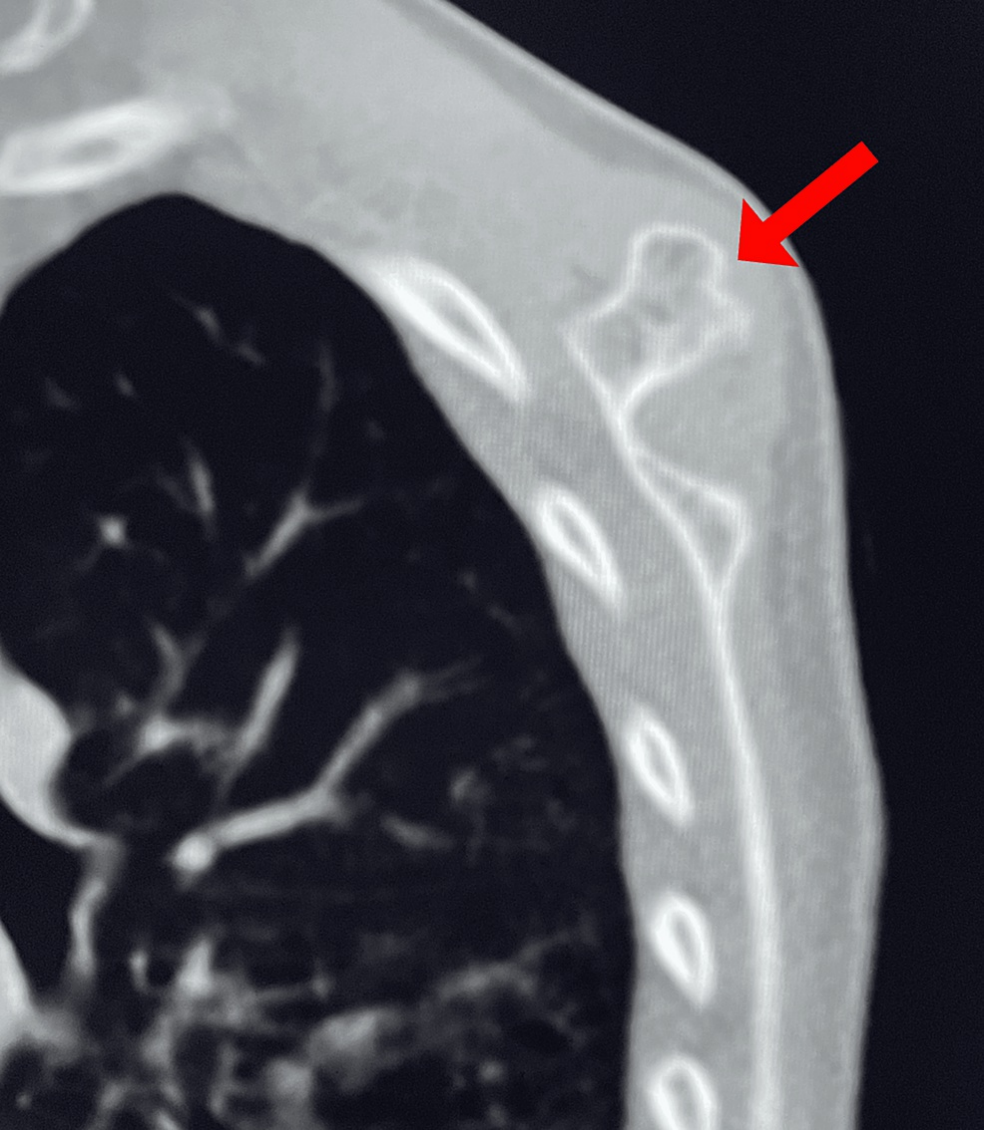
CT scan reveals abnormal bone growth. The image shows a posterior exostosis of the right scapula (arrow).
